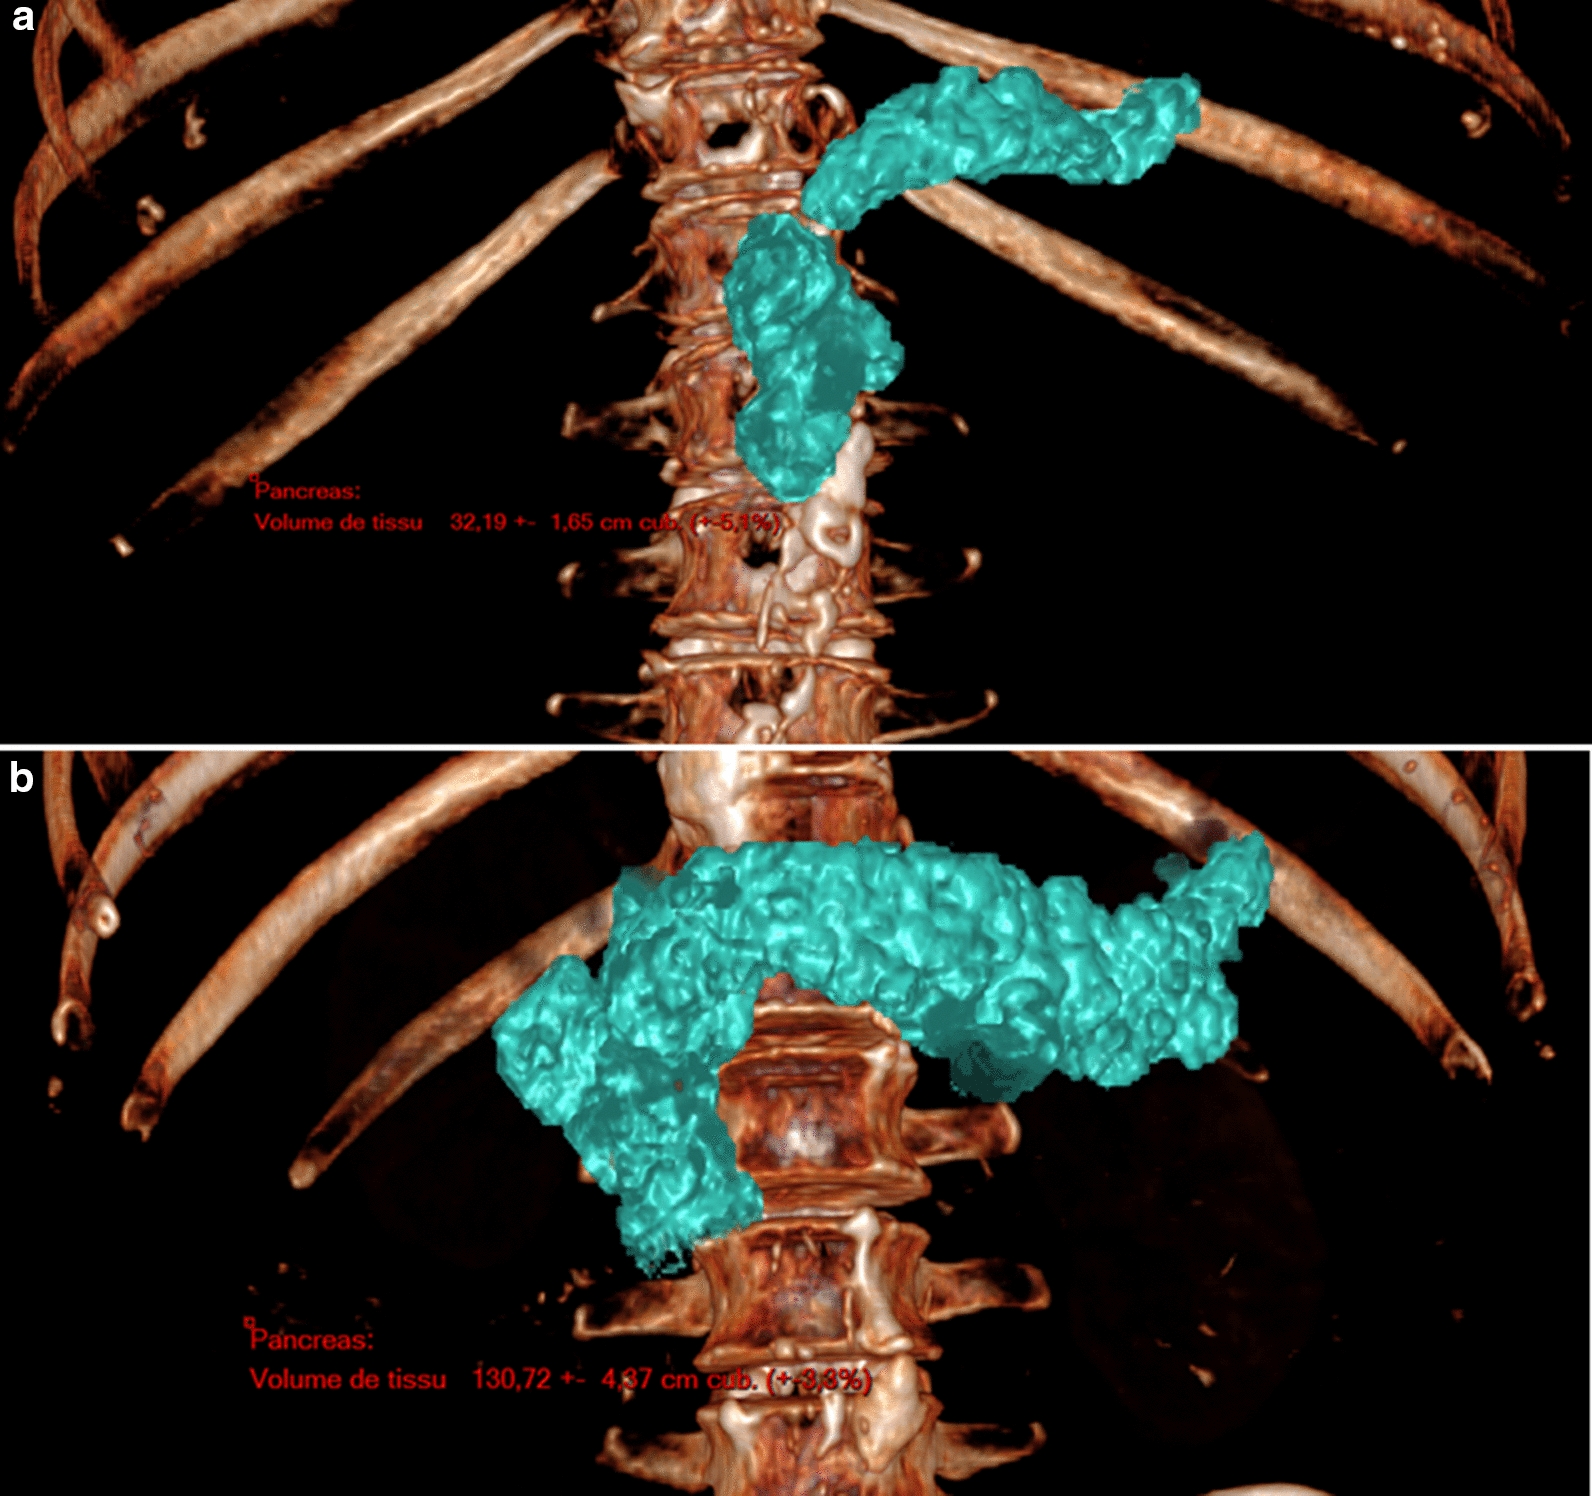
Three-dimensional computed tomography image of the pancreas. a Calculation of the pancreatic volume reveals a volume of 32 ± 1.7 cm^3^. b Calculation of the pancreatic volume reveals a volume of 131 ± 4 cm^3^
